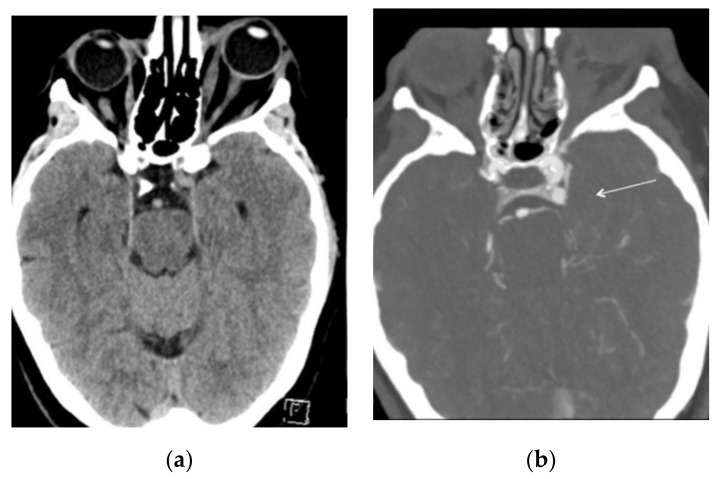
(a) CT scan on admission showing left exophthalmos with hypertrophic extrinsic ocular muscles. (b) CT angio on the same day demonstrating a small venous pouch on the posterior aspect of the left cavernous sinus (white arrow).
